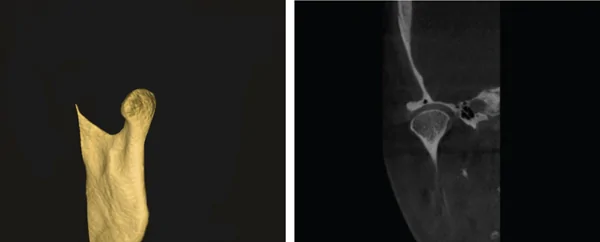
CBCT image of the left mandibular condyle showing normal single‐headed condylar morphology.
